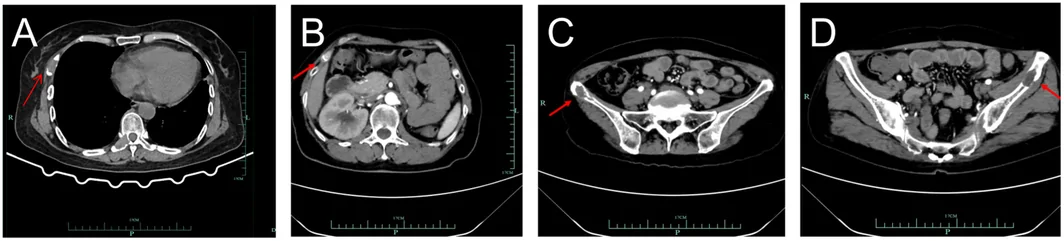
The CT scan revealed multiple metastatic lesions in the ribs (A, B) and pelvis (C, D).
